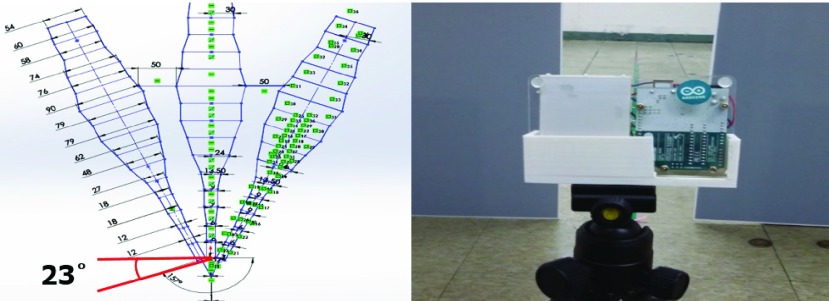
To prevent the interference of ultrasonic waves, ultrasonic profiles were made. To prevent the interference of the ultrasonic wave is manufactured by tilting 23°.
